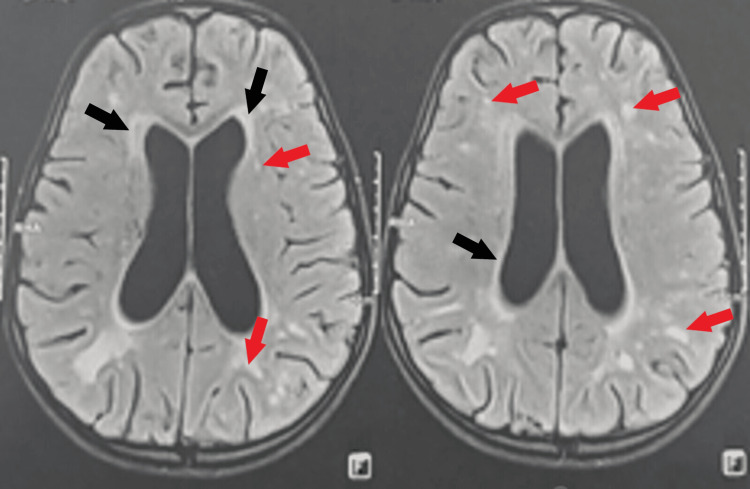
Preoperative MRI of the brain. Brain MRI in axial planes FLAIR sequence revealed moderately dilated lateral ventricles with a periventricular hypersignal, indicating its active characteristic (black arrows). Also visible are multiple lesions in hypersignal FLAIR (red arrows) located in the periventricular white substance and the right internal capsule, indicating ischemia probably due to acute demyelinating encephalomyelitis. FLAIR, fluid-attenuated inversion recovery; MRI, magnetic resonance imaging
